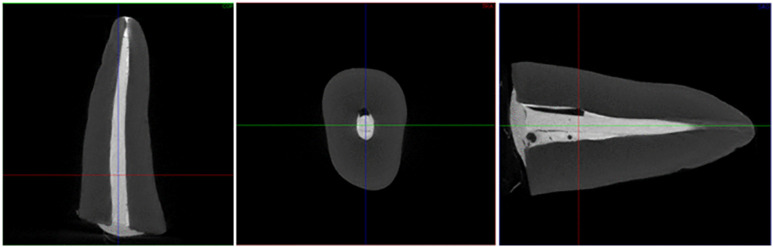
3D micro-CT images of the obturation in a sample from the Angelus Bio sealer with ultrasonic activation (UA) group. (3A) Sagittal view. (3B) Axial view. (3C) Coronal view.
